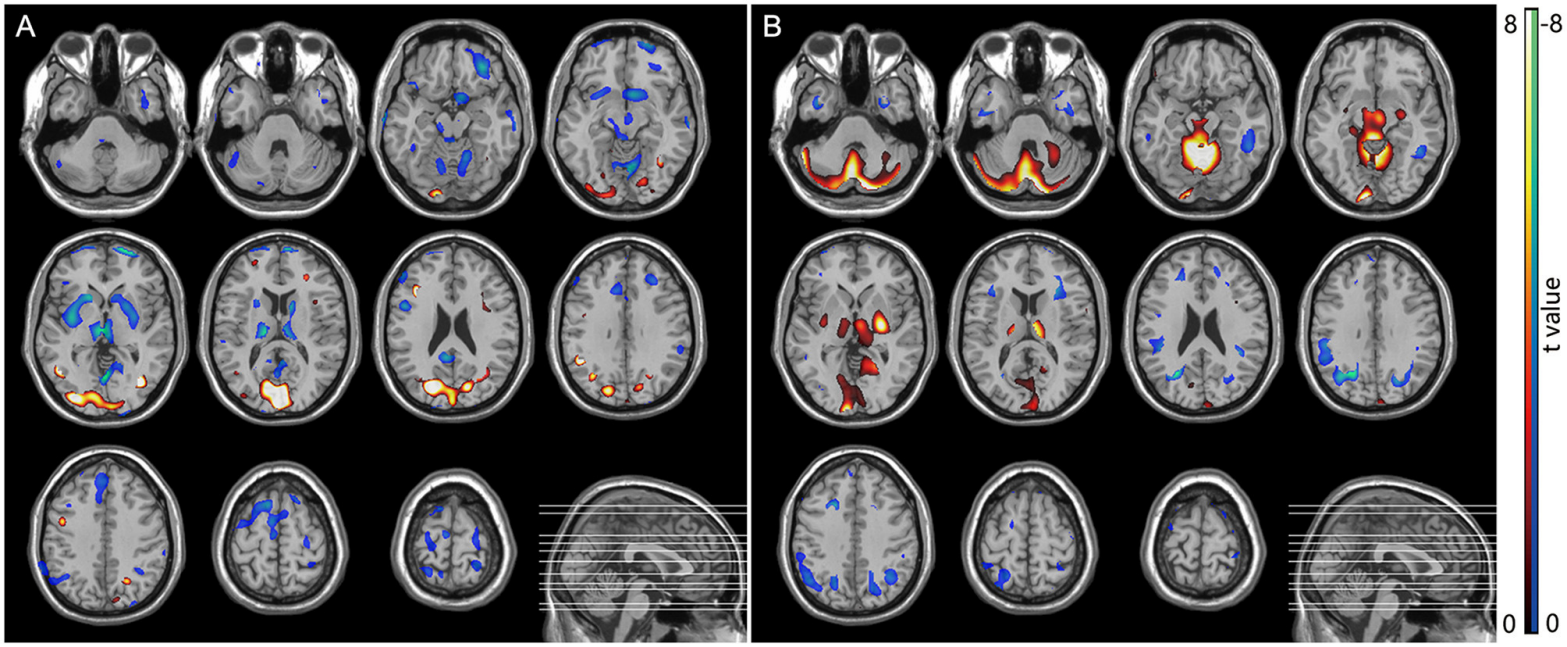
SPM analysis of 18F-FDG PET. (A) MSA patients vs. normal controls showed hypometabolism in the bilateral putamen. (B) PD patients vs. normal controls showed hypermetabolism in the bilateral putamen, cerebellum, and occipital lobes, along with hypometabolism in the frontal, parietal, postcentral cortices, and cingulate areas.
